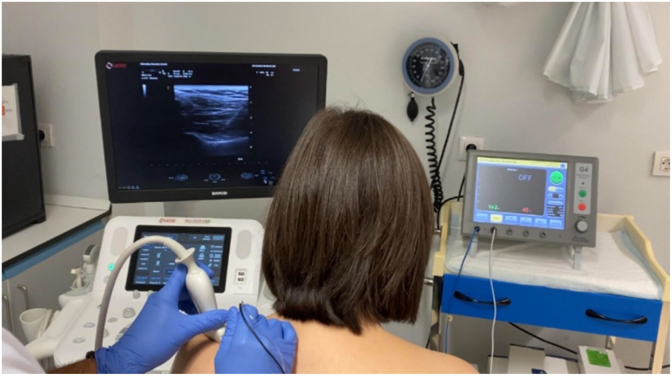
Ultrasound-guided pulsed radiofrequency of the suprascapular nerve. Authors' own images.
